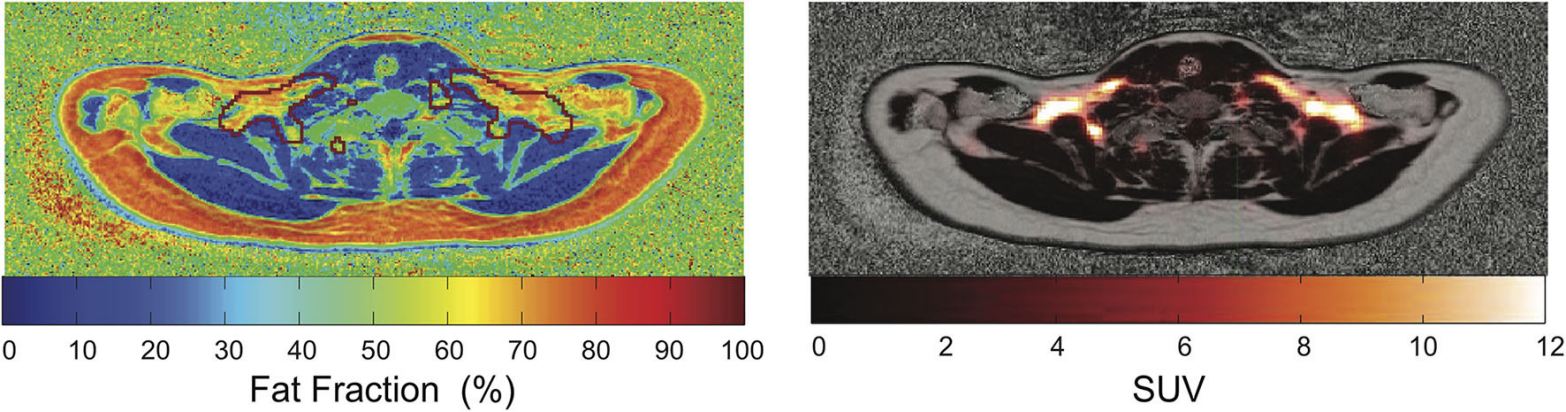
Overlay of PET images and MR-based PDFF maps. The red mark indicates regions with SUV > 2.5. This figure is original and based on data from (92).
